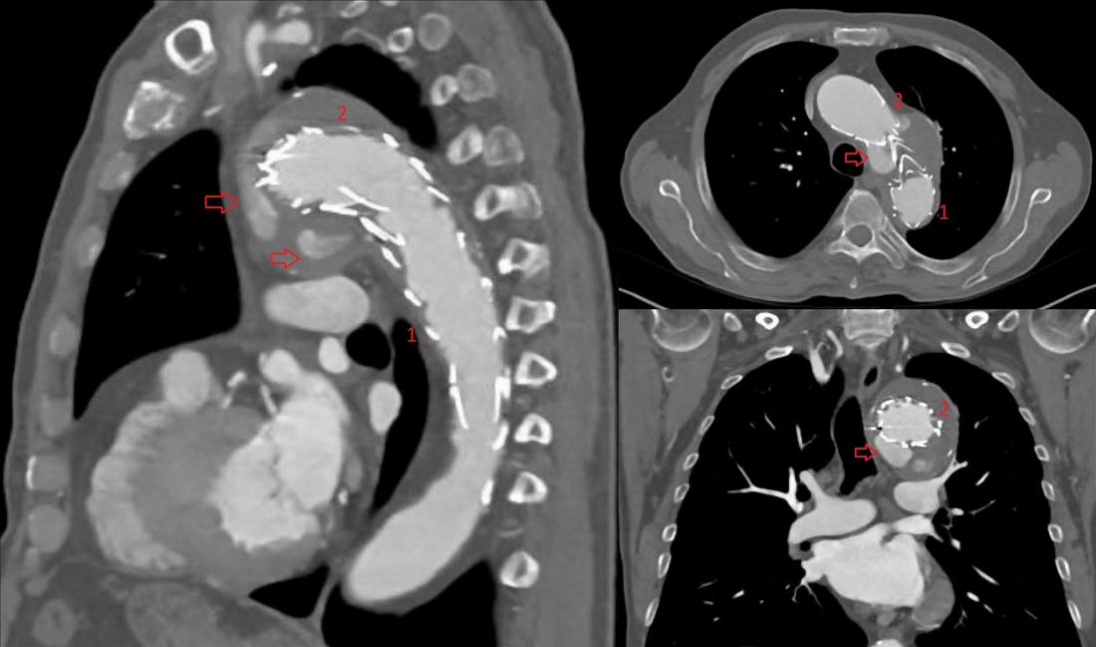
CT-scan showing a dilated aortic arch 65 × 67 mm due to persistent type 1A endoleak in zone 2, arrows. First TEVAR Medtronic Valiant Captivia 36 × 32 × 150 mm with proximal landing in zone 3, number 1; Second TEVAR Medtronic Valiant Captivia 38 × 38 × 100 mm with proximal landing in zone 2, number 2.
